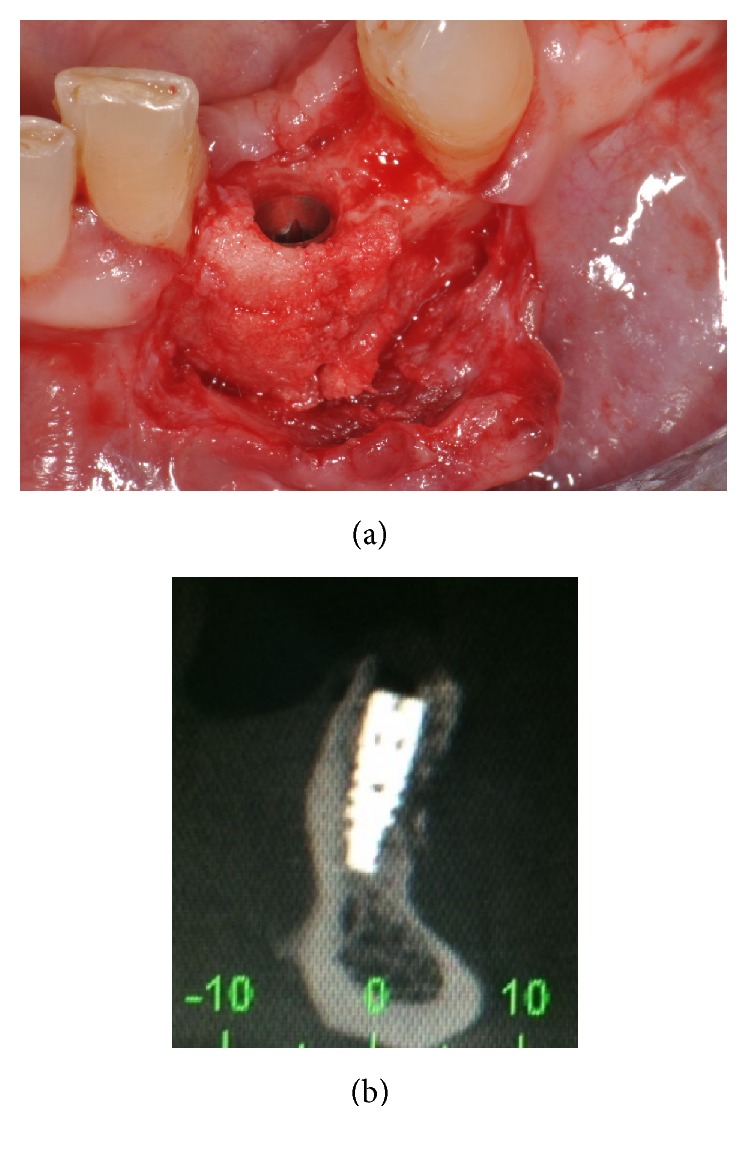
The implant was located in the perfect 3D position: (a) it was placed in a clinically well-integrated, regenerated bone; (b) as confirmed by the CBCT control examination.
